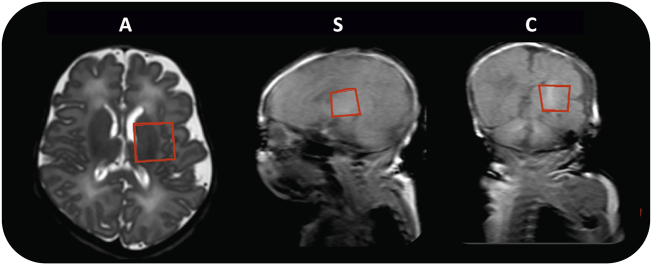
MRS acquisition region of interest. Example of region of interest size 20 × 20 x 20 mm^3^ for MRS, positioned over the left basal ganglia and thalamus. This is shown superimposed on an axial T2-weighted image (A), plus on sagittal (S) and coronal (C) T1-weighted gradient echo pilot scans.
